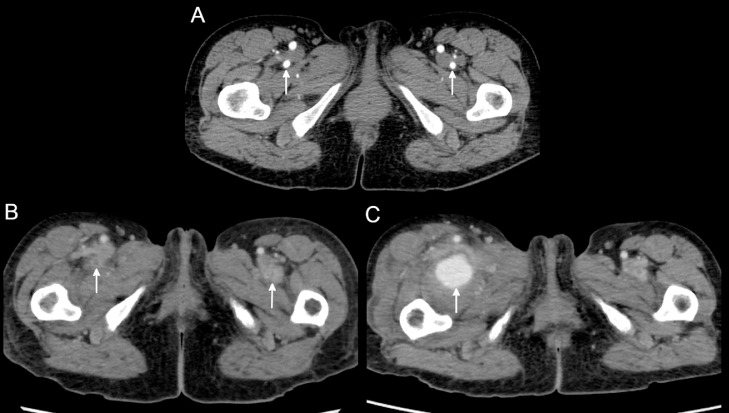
Contrast-enhanced computed tomography (CT) at the level of the deep femoral artery (DFA). (A) Contrast-enhanced CT showing normal bilateral DFAs (arrows). (B) Contrast-enhanced CT performed 1 month after (A) showed occlusion and abnormal vessel wall thickening of bilateral DFAs (arrows). (C) Contrast-enhanced CT performed 2 weeks after (B) showed a pseudoaneurysm of the right DFA (arrow).
